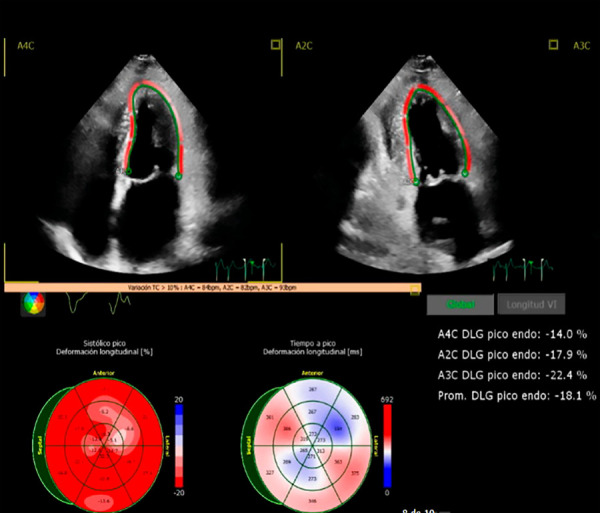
Echocardiogram showing improvement in global longitudinal strain two years after diagnosis.
